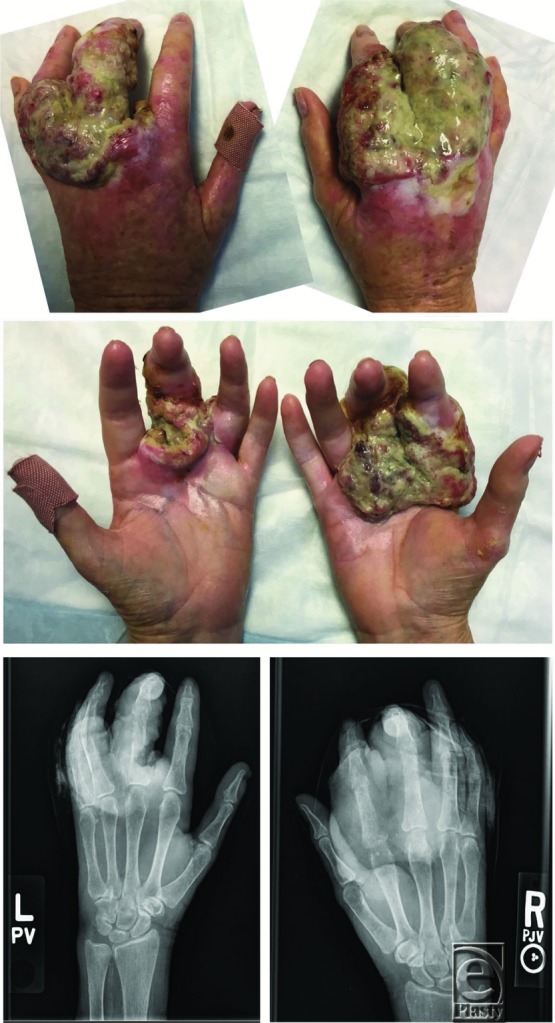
Preoperative photographs and plain films.
